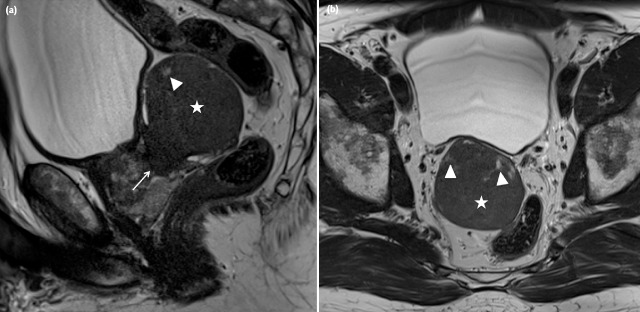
a) (sagittal T2w TSE) and b) (transverse T2w TSE). T2-weighted imaging showed a sharply demarcated structure (asterisk –5.8 × 6.5 × 5.2 cm) originating from the right part of the transition zone at the level of the midprostate (arrow) with massive posterior bulging The lesion was slightly T2 hyperintense relative to muscle (figure 1a and 1b), with small central T2 hyperintense areas (arrowhead).
